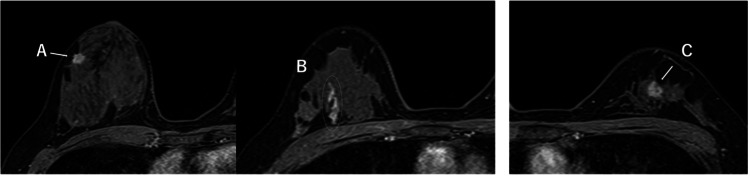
Contrast-enhanced MRI findings (A) An irregularly enhancing mass is observed at the 9 o’clock position in the right breast. (B) Non-mass enhancement is observed at the 11 o’clock position in the right breast. (C) An irregularly enhancing mass is observed at the 12 o’clock position in the left breast MRI: magnetic resonance imaging
